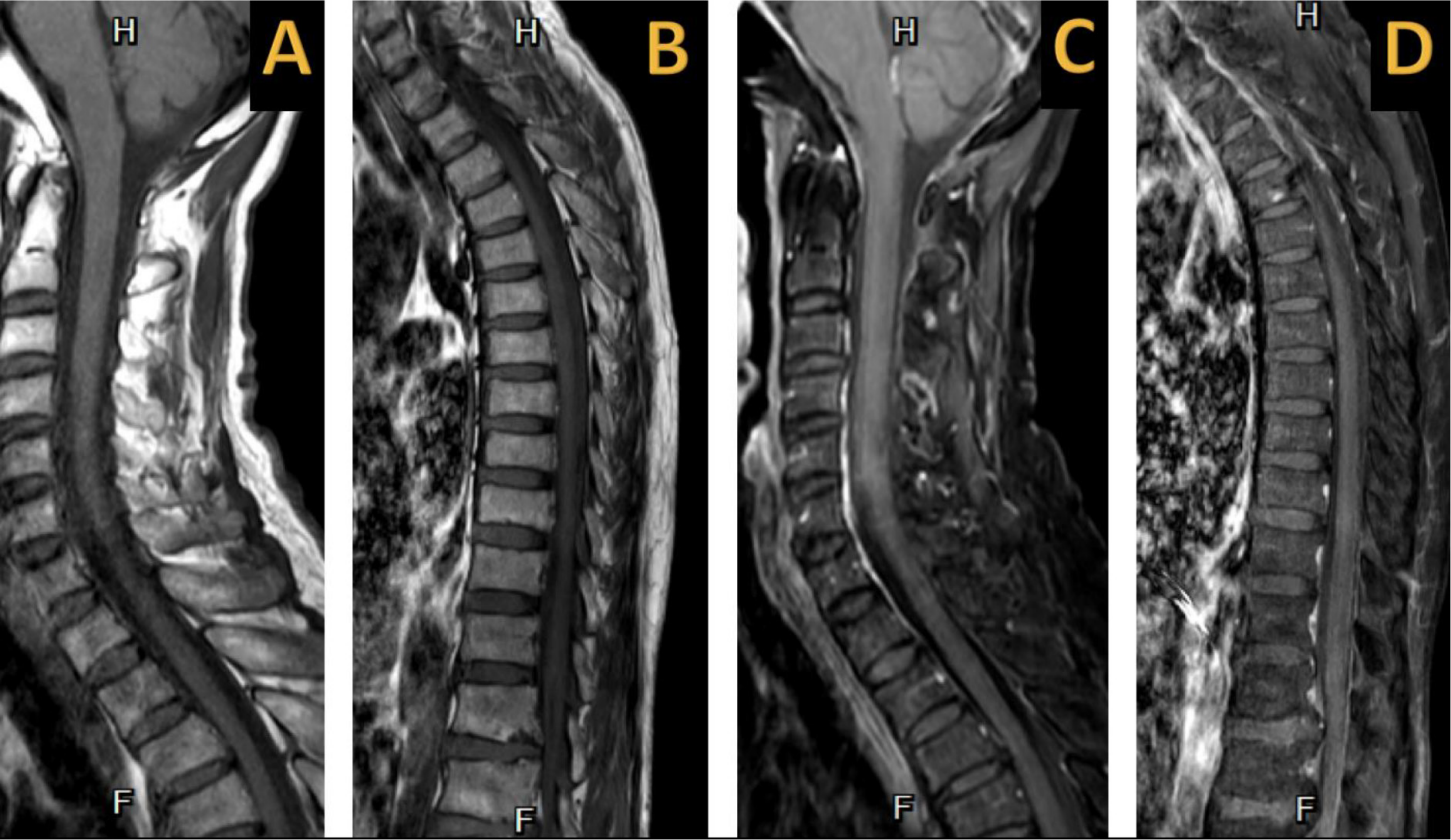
T1-weighted (A, B) and T1-weighted with gadolinium (C, D) spinal magnetic resonance imaging depicts the absence of abnormal signal intensity and gadolinium enhancement.
